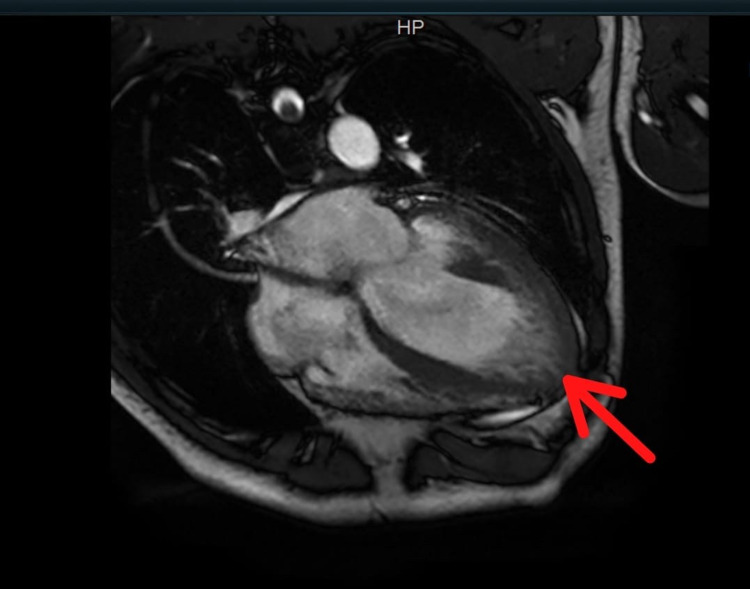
Cardiac MRI revealed a severely dilated left ventricle with increased trabeculations within the mid to apical inferior, mid to apical lateral and true apex (highlighted by the red arrow)
